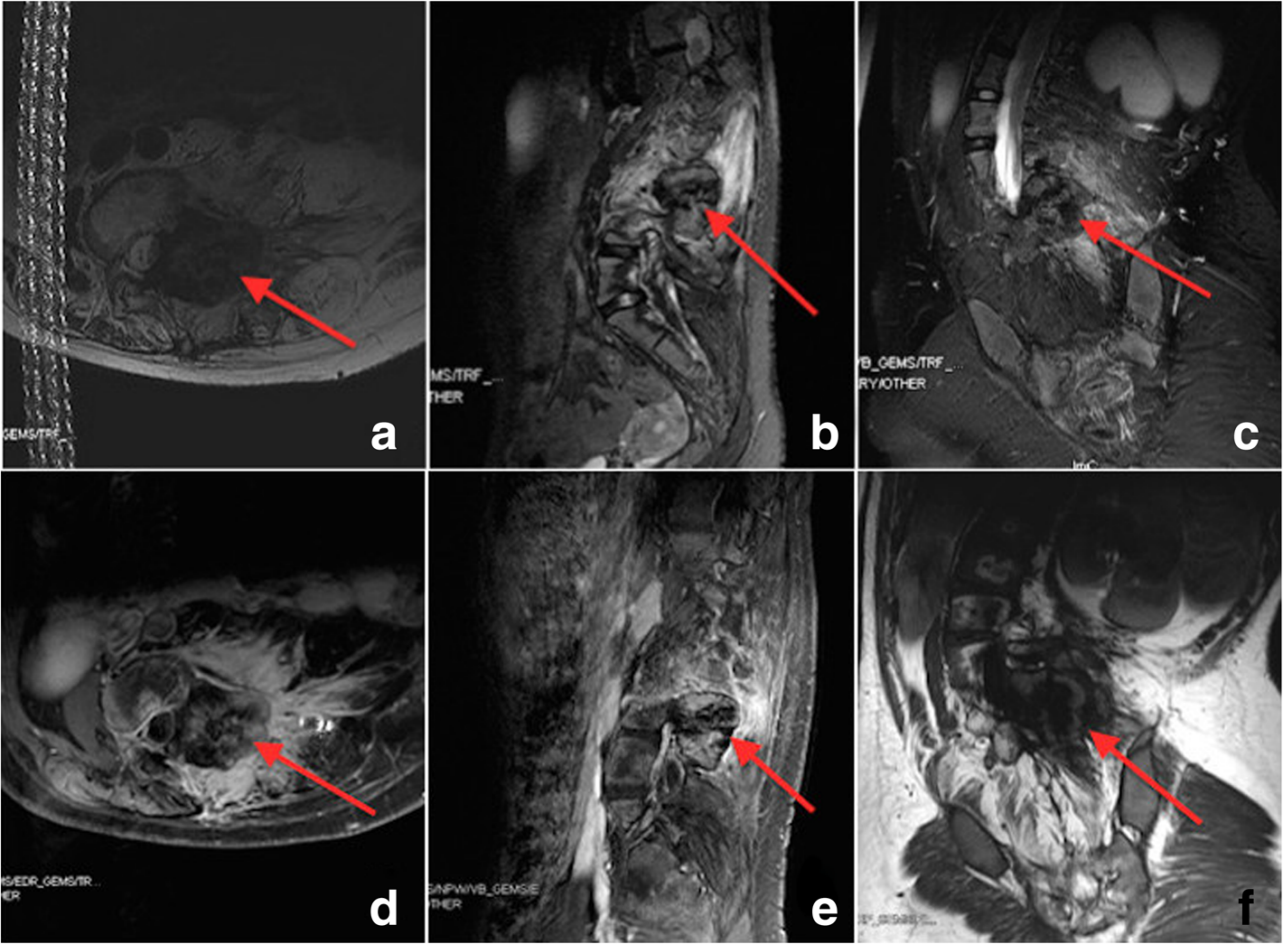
Contrast-enhanced magnetic resonance image of the lumbar spine demonstrated a heterogenic characteristic signal consisting of high and low signal intensities on both T1- and T2-weighted images (red arrow). Axial MRI showed narrowed spinal canal. (a, d) transaxial, (b, e) sagittal, (c) coronal T1-weighted images, (f) coronal T2-weighted images
